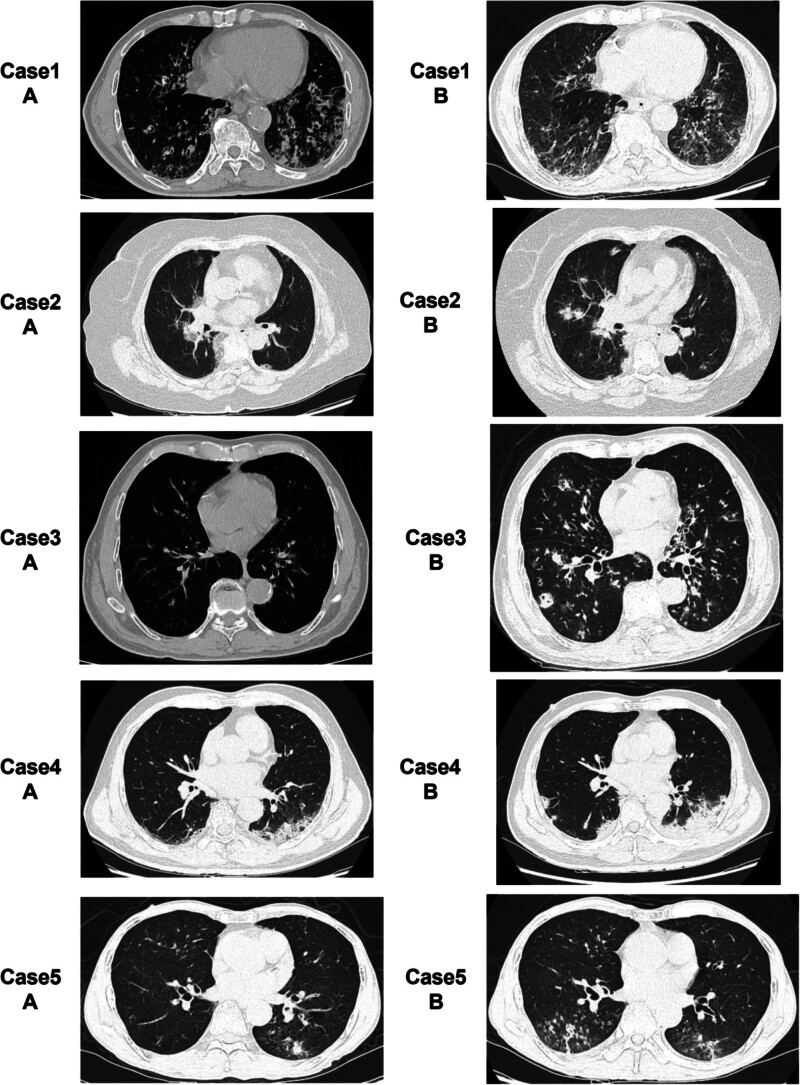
Initial (A series) and subsequent CT (B series, taken after 5–10 days of antiviral treatment and before initiating of antifungal therapy) CT images of patients with influenza and *Aspergillus* co-infection are shown. CT = computed tomography.
